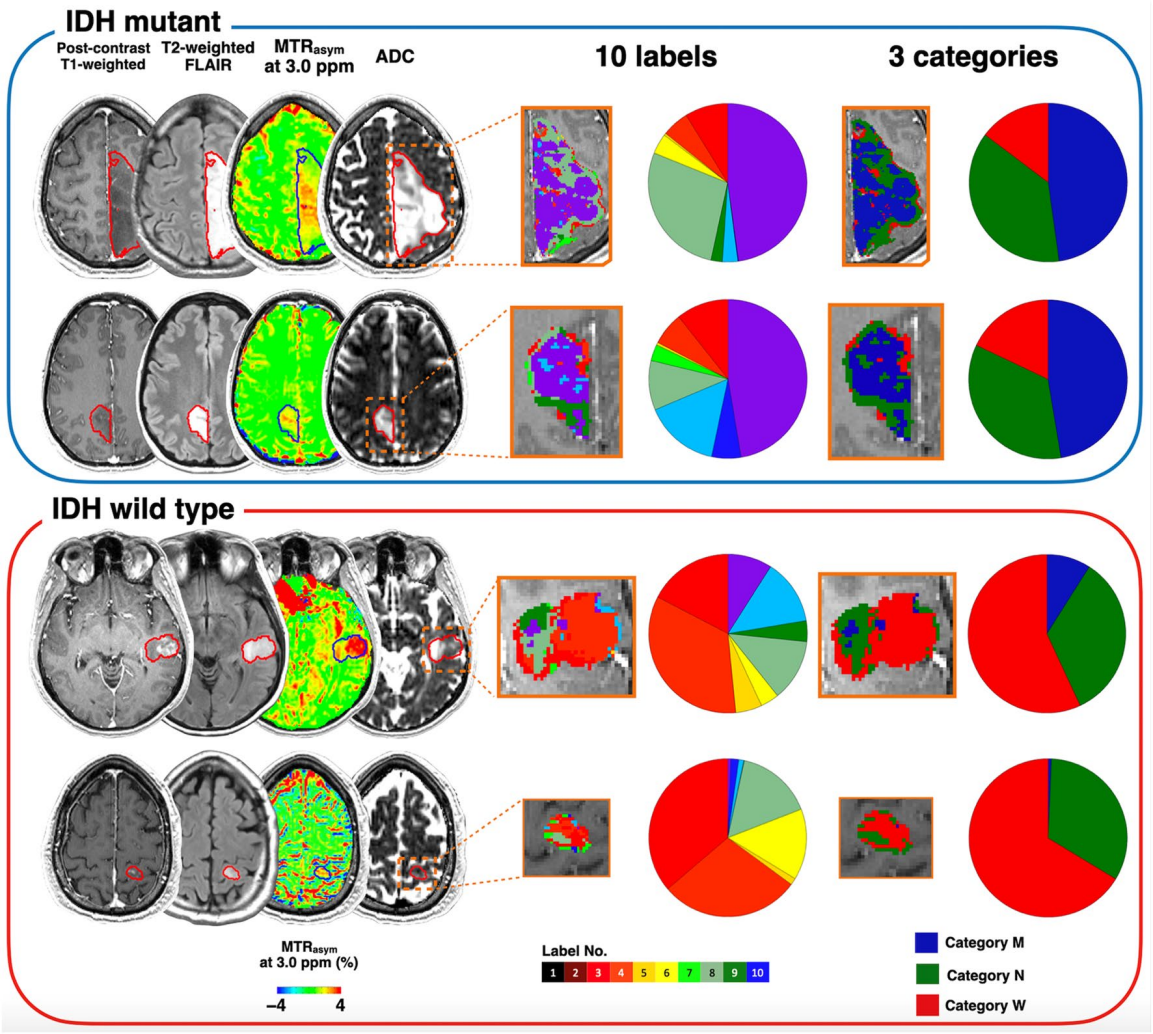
Representative cases of IDH mutant and wild-type gliomas with 10-class clustering. The CE-T1WI and FLAIR images, MTR_asym_ at 3.0 ppm, and ADC maps are shown for each patient. Each color within the tumor ROIs corresponds to each label in the 10-color bar and each category in the 3-color bar. The ratios of each label and category are shown in pie charts. In these examples, labels in category *M* (label 1) occupied about half of the tumor ROIs in IDH mutant gliomas, while labels in category *W* (label 7–10) occupied less than a quarter of the tumor ROIs. In IDH wild-type gliomas, labels in category *W* occupied the majority of the tumor ROIs.
